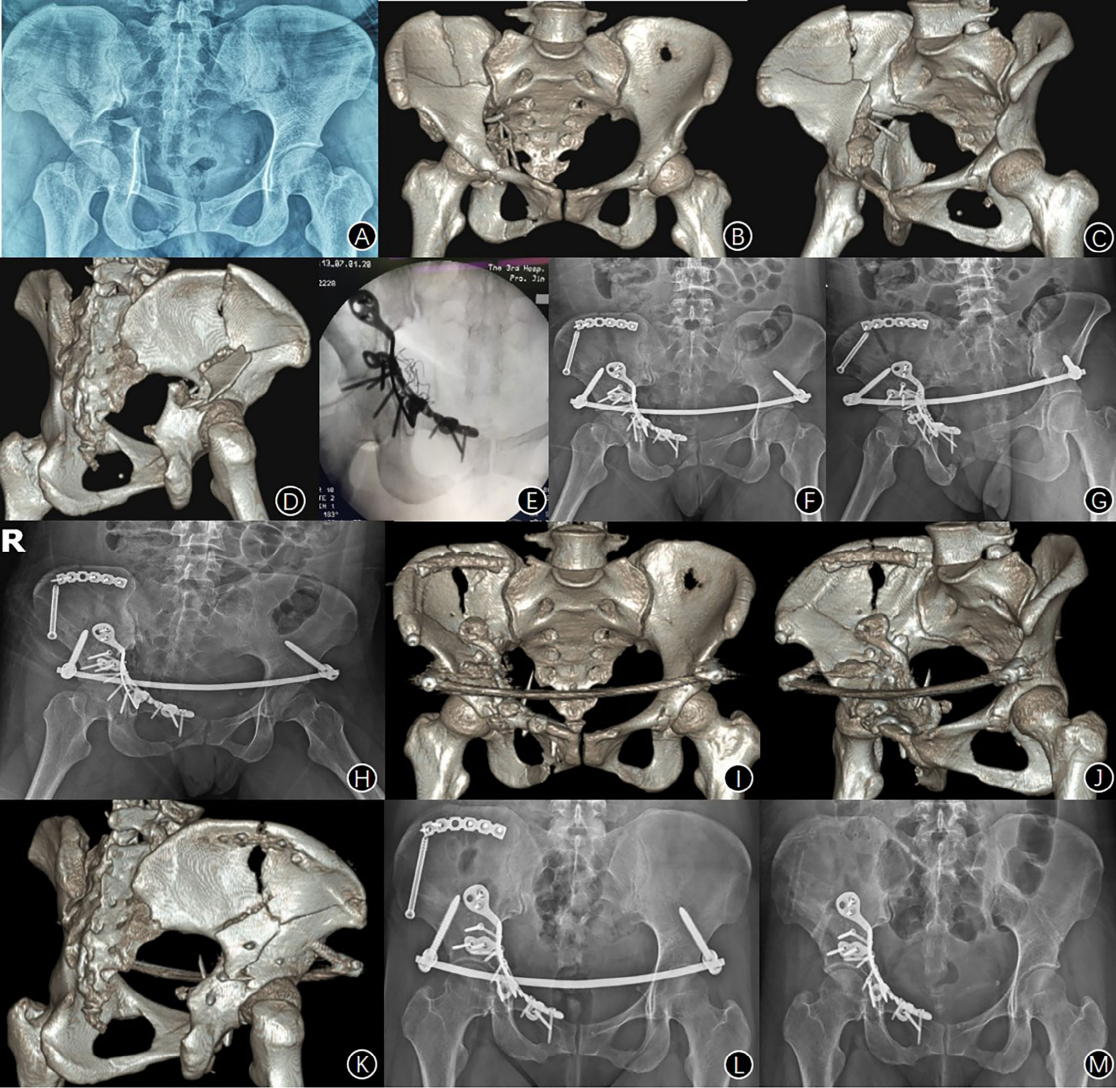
A 36‐year‐old female with fresh acetabular both‐column fracture, who had been admitted to the hospital 2 h after the traffic injury, was treated with an AIAP via the LRA. (A) Preoperative radiograph of anteroposterior view; (B–D) Preoperative three‐dimensional reconstructions; (E) Intraoperative radiograph of anteroposterior view; (F–H) Postoperative radiograph of anteroposterior, iliac oblique and obturator oblique views; (I‐K) Postoperative three‐dimensional reconstructions; (L) Anteroposterior radiograph at 3 months after operation; (M) Anteroposterior radiograph at 1 year after operation.
